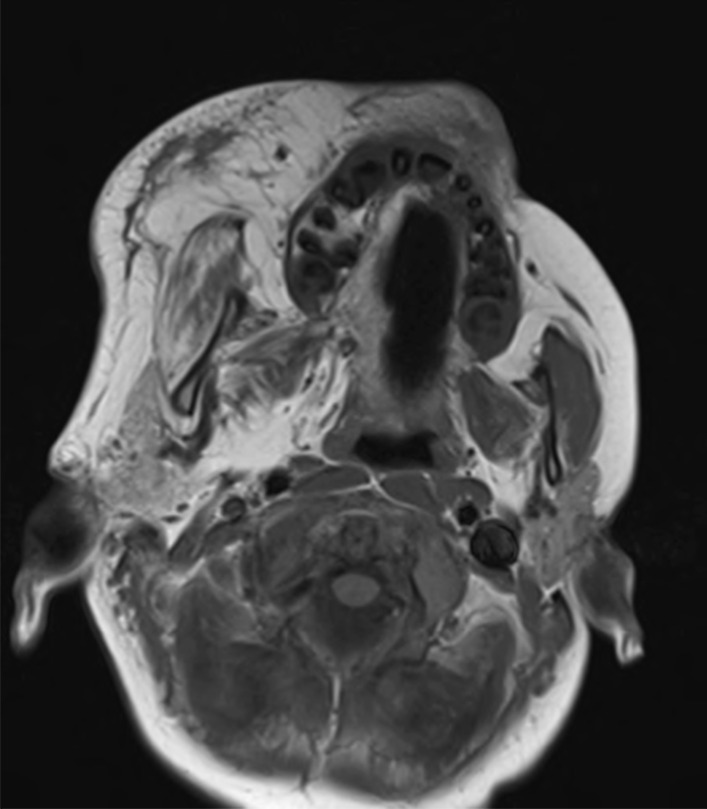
MRI at 4 years demonstrating extensive fatty infiltration and hypertrophy of the right side of the face (patient two)
